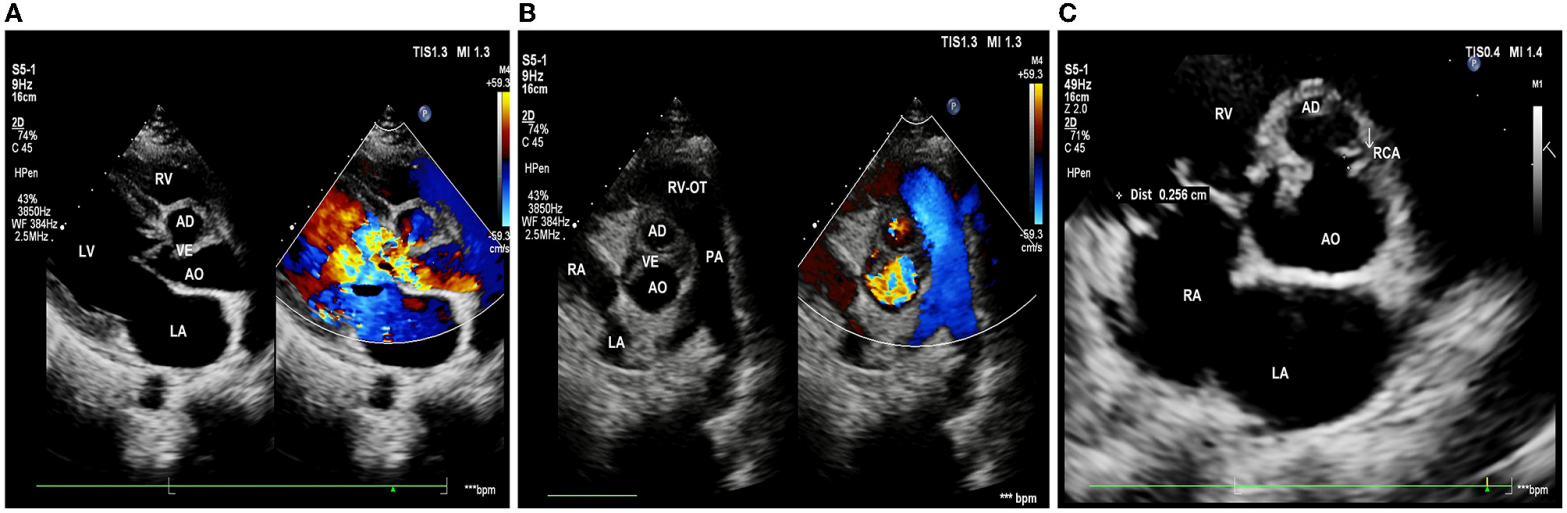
Pre-operative echocardiography. (A) Long-axis view of the left ventricle shows aortic valves thickening and flail motion, and color Doppler shows massive aortic regurgitation. (B) Short-axis view of the great arteries shows that the bulge in the aortic root communicates with the aorta, with vegetations in the aortic valve. (C) The right coronary artery (white arrow) is restrictive; LA, left atrium; LV, left ventricle; RV, right ventricle; AO, aorta; RVOT, right ventricular outflow tract; PA, pulmonary artery; RCA, right coronary artery; AD, aortic disease; and VE, valvular excrescence.
